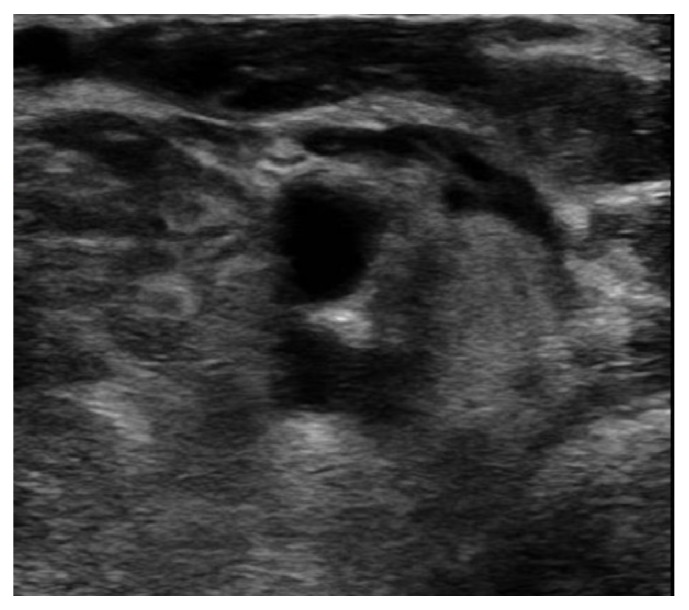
Venous ultrasound with Doppler of left upper extremity showing findings suggestive of a nonocclusive DVT of proximal/mid left subclavian vein which is difficult to compress.
